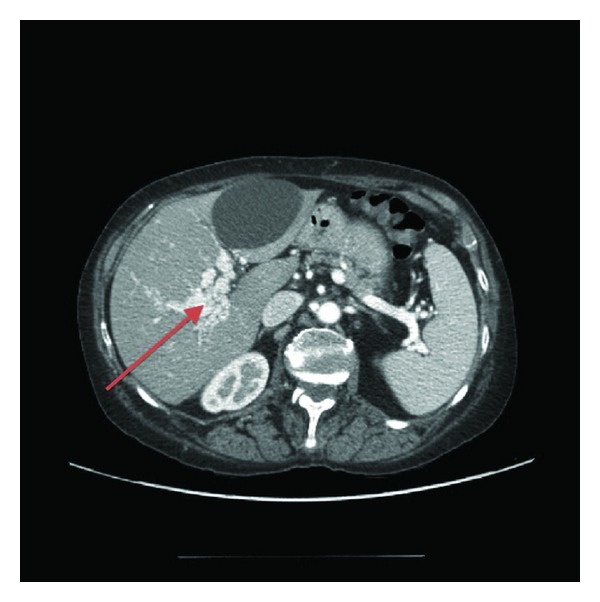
Residual portal cavernoma showed at CT scan.
